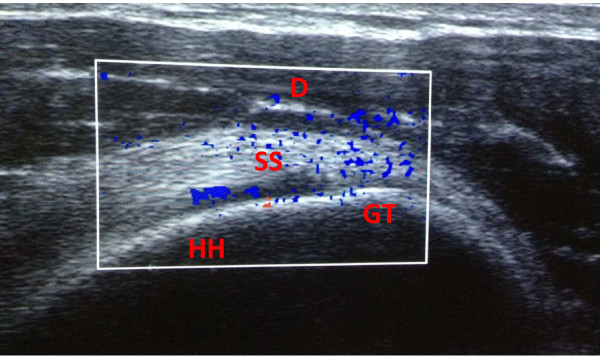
Power Doppler US imaging shows vessels mainly at supraspinatus tendon during examination of volleyball player’s shoulder. Supraspinatus tendon (SS), greater tuberosity (GT), humeral head (HH) and deltoid muscle (D) are labeled. The oximetry value was 63%.
